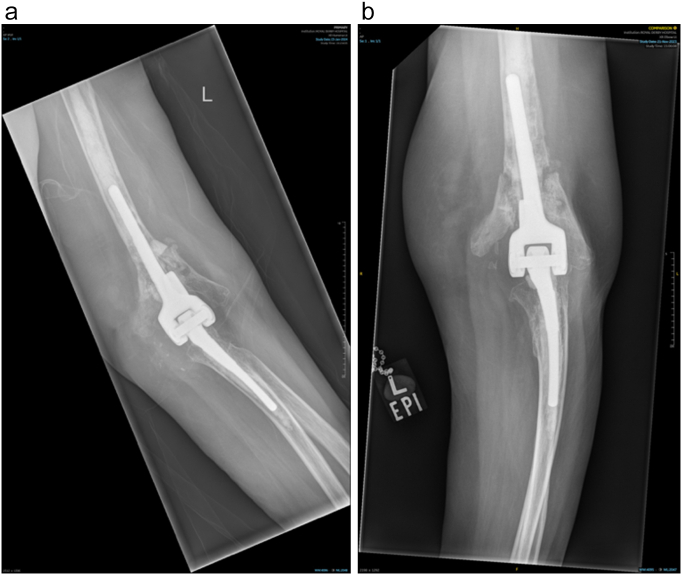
Anteroposterior [A] and lateral [B] radiographic views showing the initially revised TEA of the left elbow on the last radiographic assessment due to loosening and periprosthetic fracture, in 2023.
